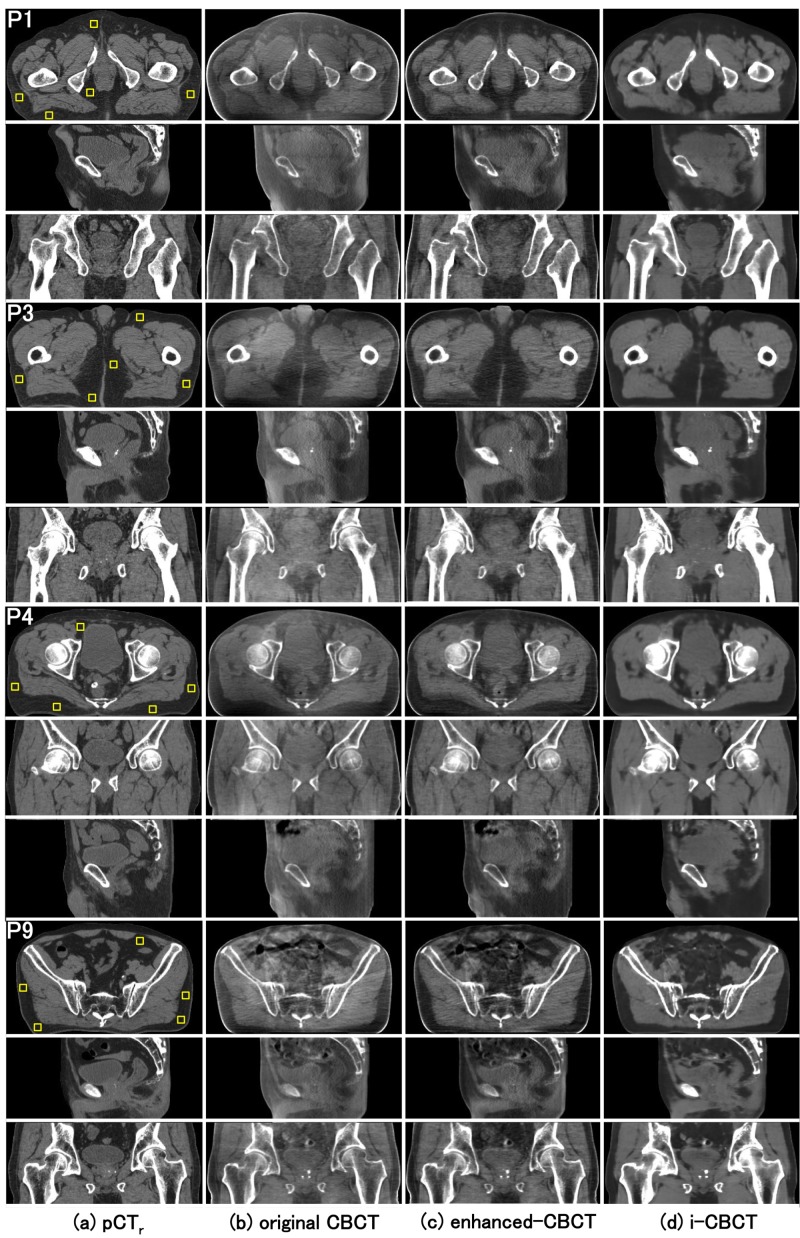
Comparison of the image qualities for representative four cases (patient 1, 3, 4, 9). For each patient, the images in the top, middle, and bottom row are axial, sagittal and coronal views, respectively. Columns (a), (b), (c) and (d) are pCT_r_, original CBCT, enhanced CBCT and i-CBCT, respectively. The yellow squares placed on the axial view of the column (a) were selected ROIs for the SNU calculation. The ROIs were set on the fat region for each patient. Display window range was set to (-400, 100) HU for the original CBCT and (-300, 200) HU for the pCT_r_, the enhanced CBCT and the i-CBCT. pCT_r_: Registered planning computed tomography; original CBCT: Original cone beam computed tomography; enhanced-CBCT: Enhanced cone beam computed tomography; i-CBCT: Improved cone beam computed tomography; SNU: Spatial nonuniformity; ROI: Region of interest.
